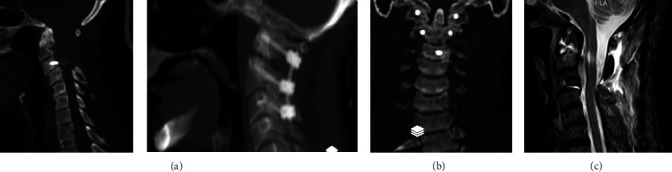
Postoperational midsagittal (a), coronal (b), parasagittal (c) cervical spine CT-scan and midsagittal T2 weighted cervical spine MRI, showing complete reduction and fusion of odontoid fracture. CT, computed tomography; MRI, magnetic resonance imaging.
